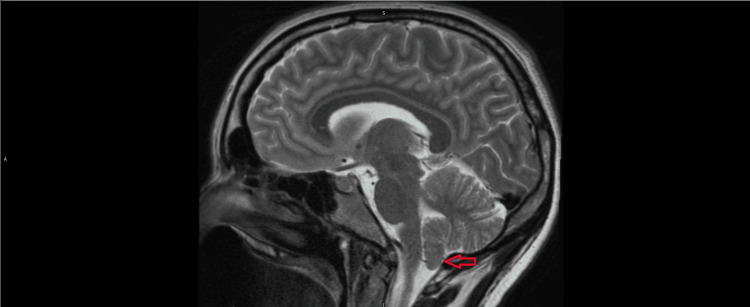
Sagittal T1-weighted MRI of the brain in a patient with Chiari Malformation Type 1 Sagittal MRI demonstrating inferior displacement of the cerebellar tonsils below the level of the foramen magnum (indicated by red arrow), consistent with Chiari Malformation Type 1. The posterior fossa structures are crowded, but there is no evidence of Syringomyelia or hydrocephalus.
